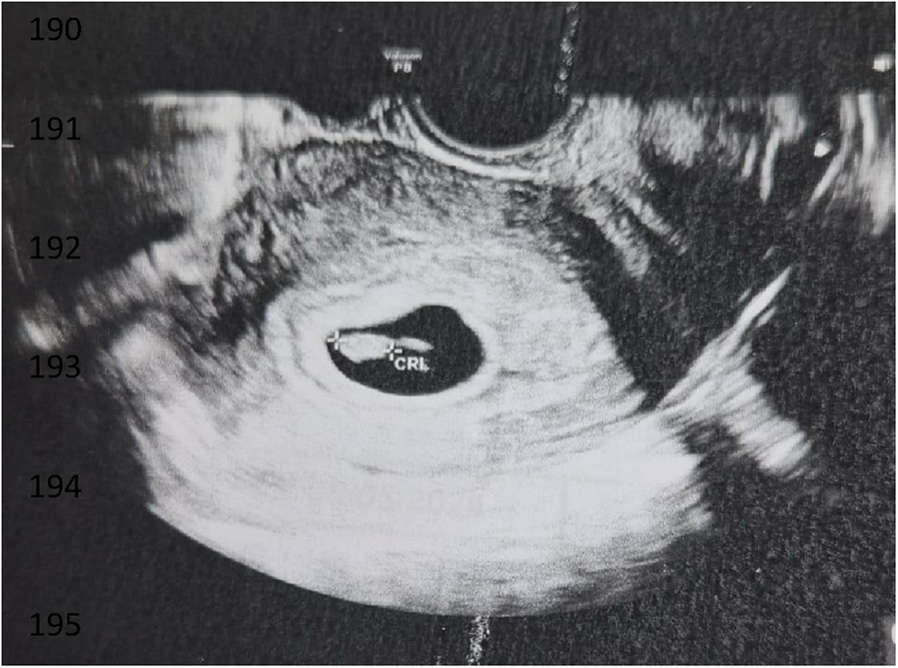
Ultrasound scan performed at 7 weeks of gestation.
